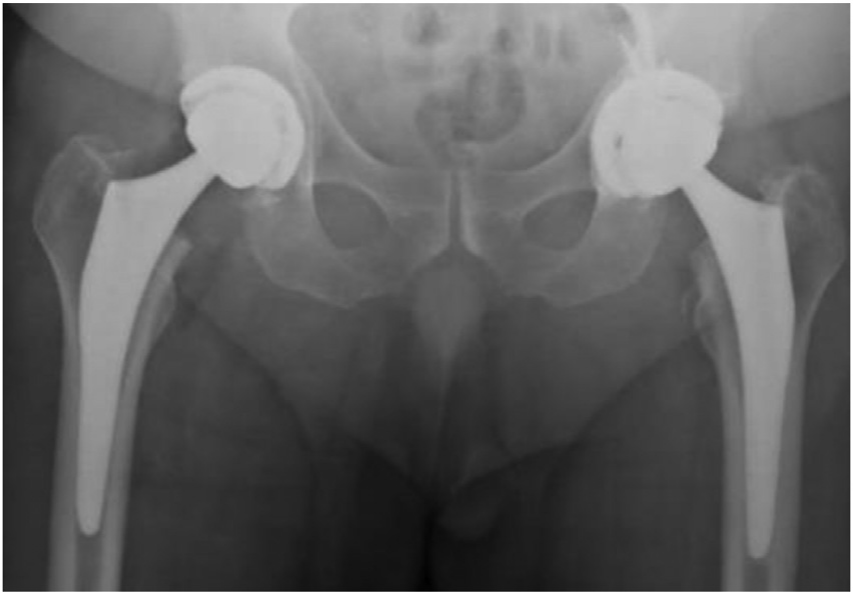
Bilateral THA postoperative radiograph. Anteroposterior (AP) pelvis radiograph demonstrates well-positioned bilateral THA without evidence of complications, fractures, or mechanical hardware failure immediately after right THA and 18 months after left THA.
